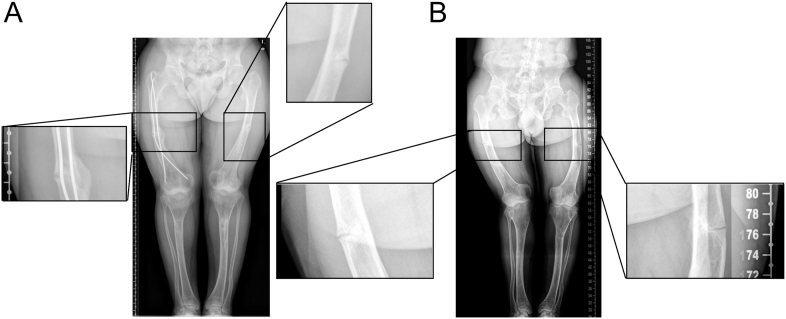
Radiographs of 26-year-old twin 1 (A) and twin 2 (B). The insets highlight diaphyseal lucencies with periosteal reaction, consistent with insufficiency fractures (pseudofractures) due to long-standing untreated hypophosphatemic rickets.
